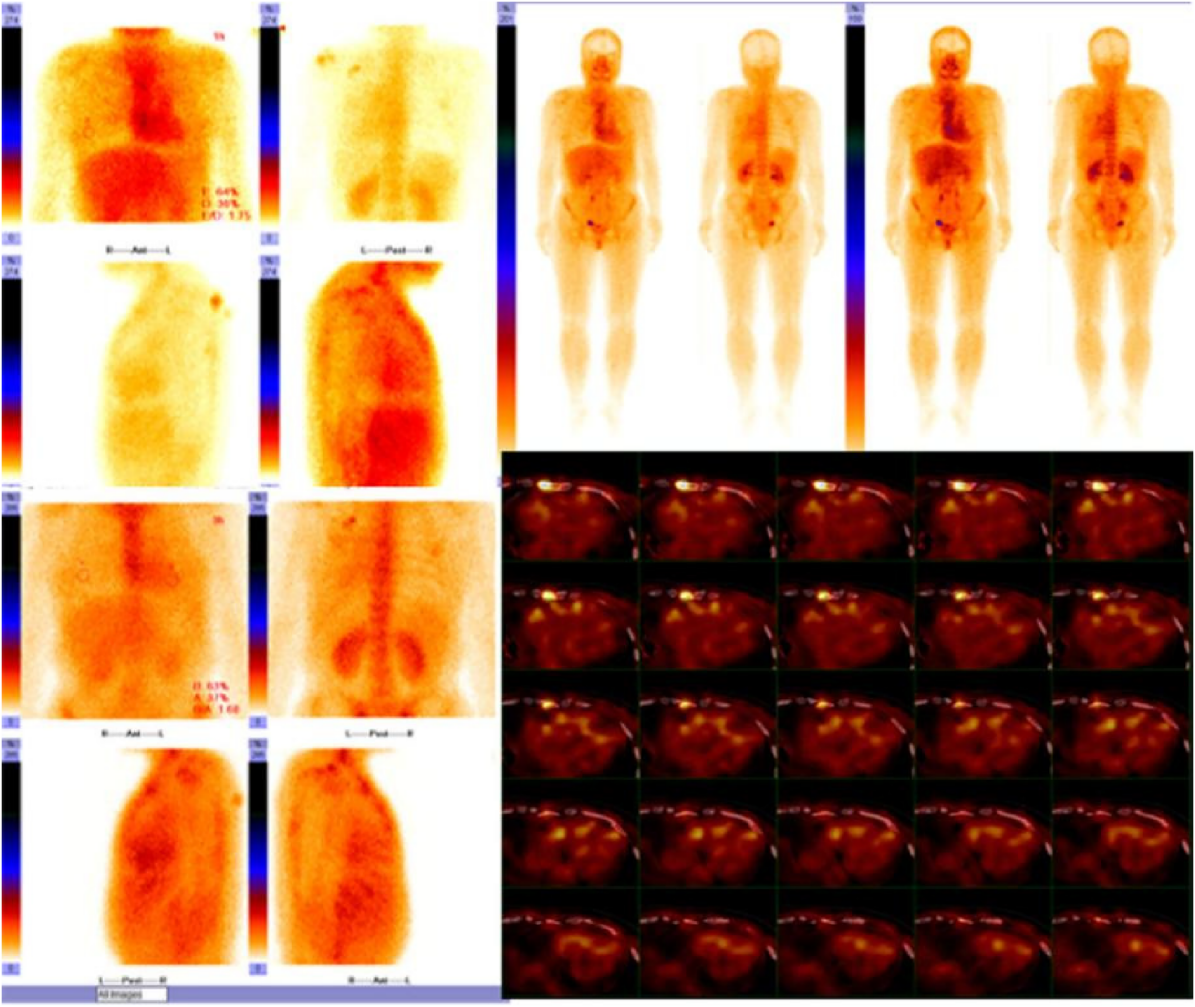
Cardiac uptake in bisphosphonate scintigraphy showing Perugini grade 2 and similar myocardial and bone uptake.
